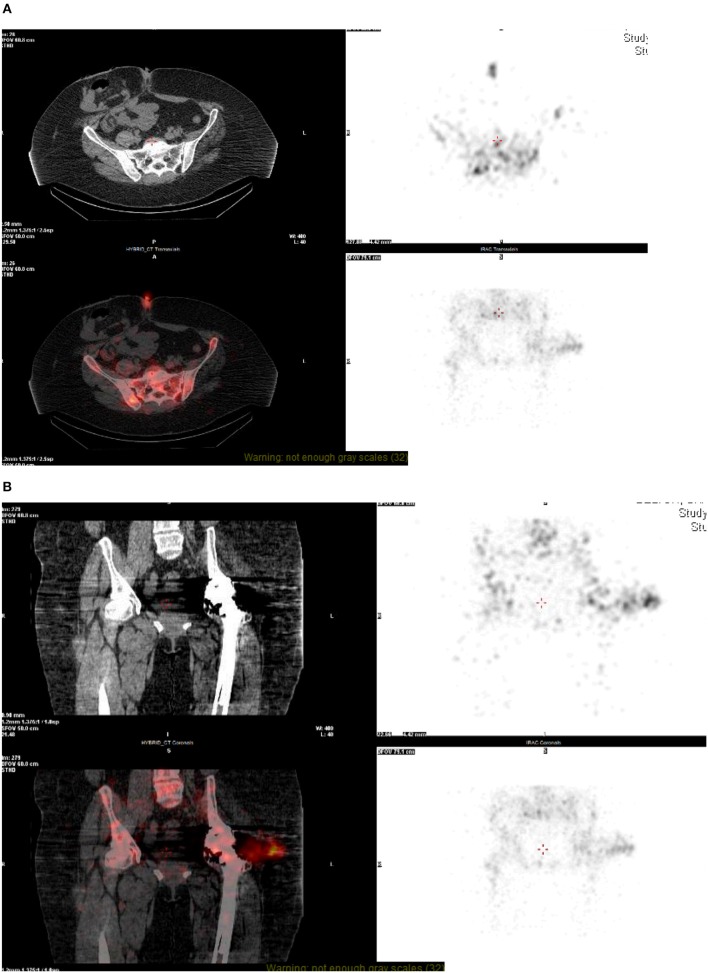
(A,B) NM technetium and white cell scan—SPECT CT. On SPECT-CT, there is image degradation seen at the level of the hip joint, despite this, there is some fluid visible lateral to the hip joint which co-localizes to the intense focus of white cell uptake. This fluid appears to track from the hip joint into the deep subcutaneous tissue, representing bursitis, or soft-tissue abscess formation. A focus of uptake can also be seen at the skin surface at the level of the umbilicus, most likely related to recent surgery.
